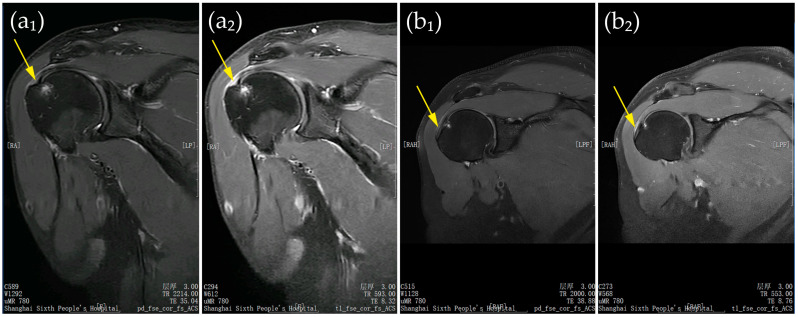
(a,b) Representative shoulder MRI scans of two patients at baseline. Panels (a_1_,b_1_) show proton density–weighted fast spin-echo images in the coronal plane with fat saturation and autocalibration for parallel imaging; panels (a_2_,b_2_) show T1-weighted fast spin-echo images in the same plane with identical parameters. Yellow arrows indicate partial-thickness ST tears. All patient-identifying information has been removed to protect privacy.
